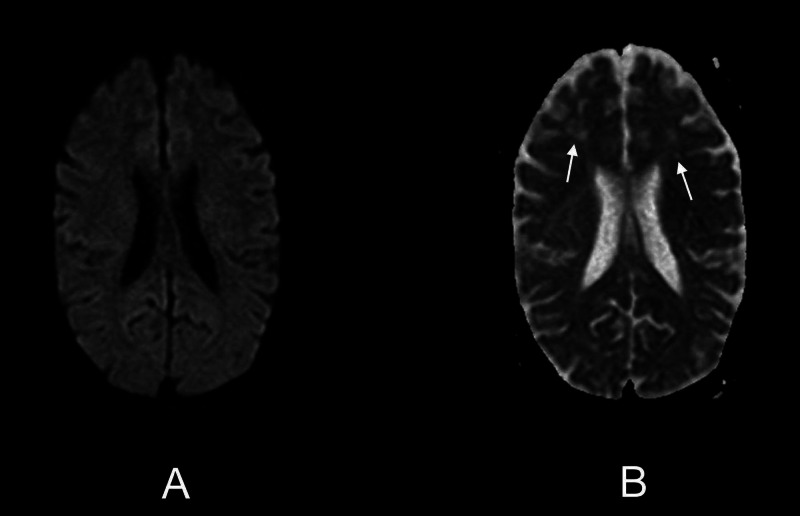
Axial MR images showing DW and ADC sequences Multiple foci are noted distributed bilaterally in the frontoparietal regions. No diffusion restriction is demonstrated on (A) axial DW MR image and (B) axial ADC MR image (white arrows). MR, magnetic resonance; DW, diffusion-weighted; ADC, apparent diffusion coefficient.
